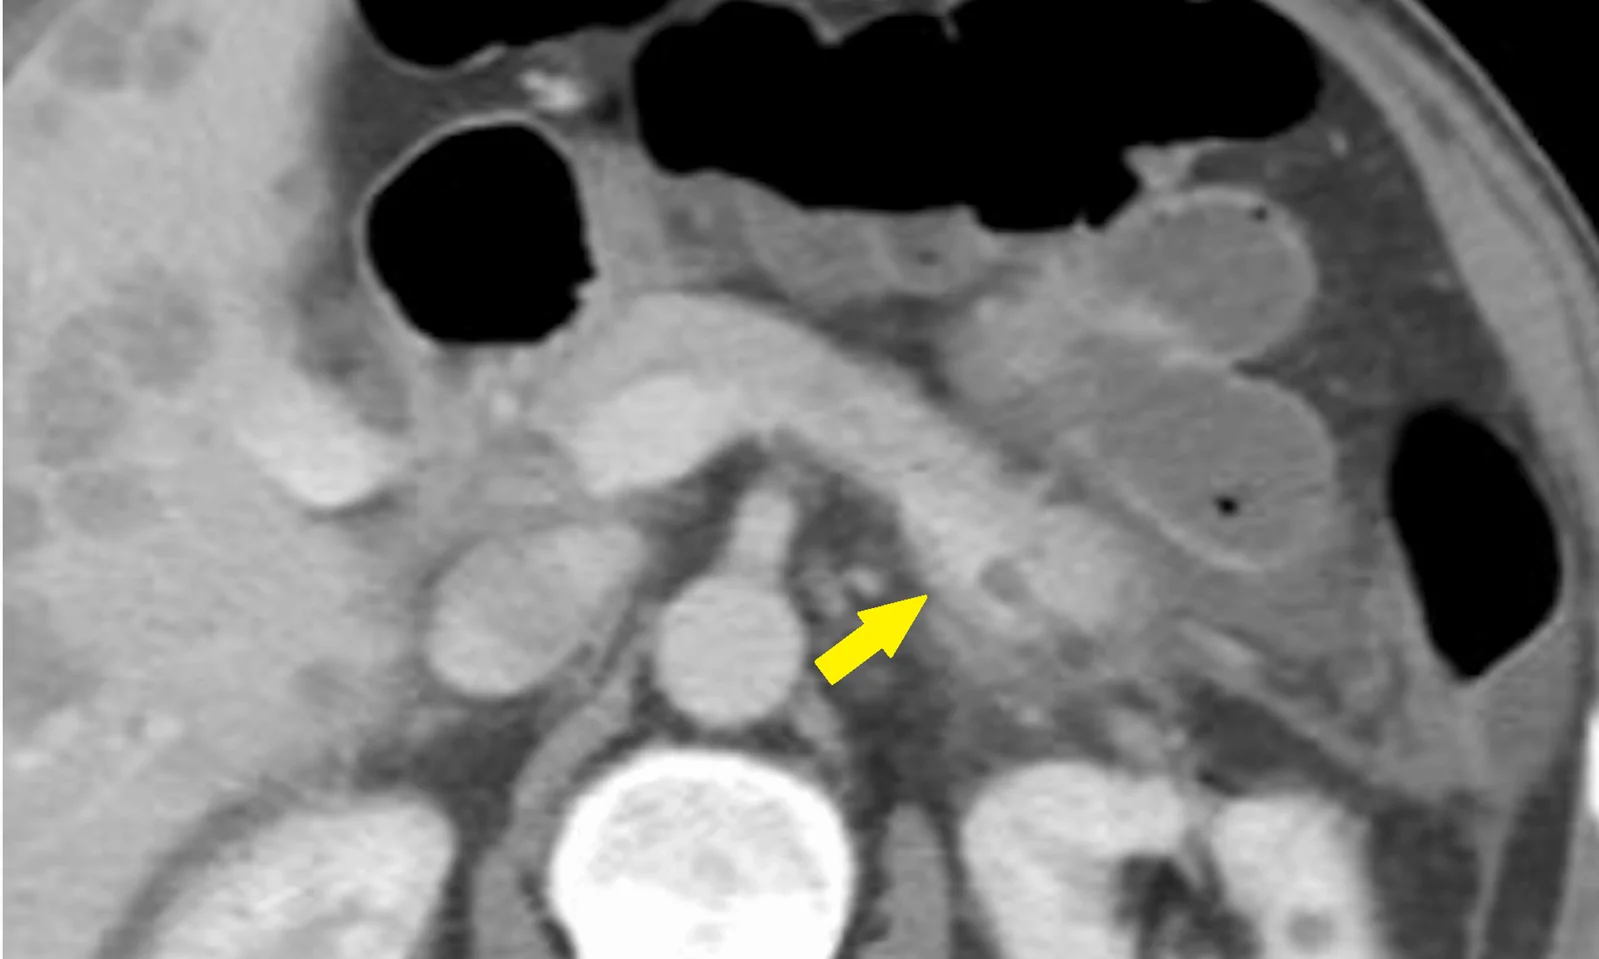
CT scan of the abdomen demonstrating splenic vein thrombosis Thrombosis of the splenic vein indicated by the yellow arrow.
